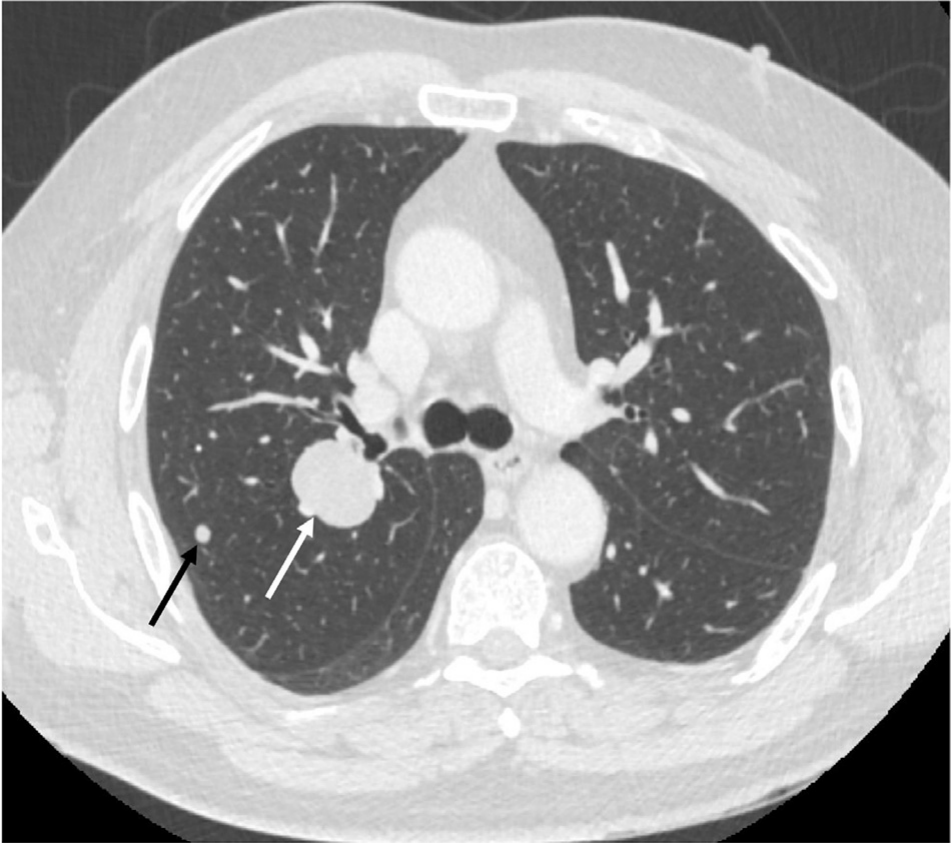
Selected thoracic CT axial slice (lung window, W:1500, L:-600) in a 58-year-old male presenting with a 3-month history of cough. The image demonstrates a 33 mm mass (white arrow) and a peripheral solid nodule in the posterior segment of the right upper lobe (black arrow). Multiple further bilateral pulmonary nodules were also identified (not shown).
